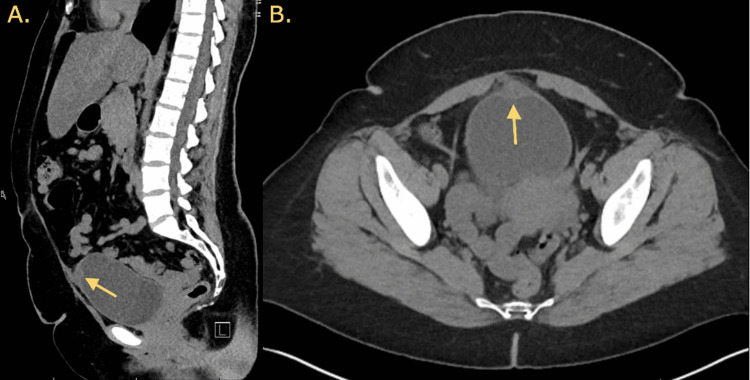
Findings of the CT of the abdomen and pelvis. A. Sagittal view of the CT of the abdomen and pelvis. B. Axial view of the CT of the abdomen and pelvis.
